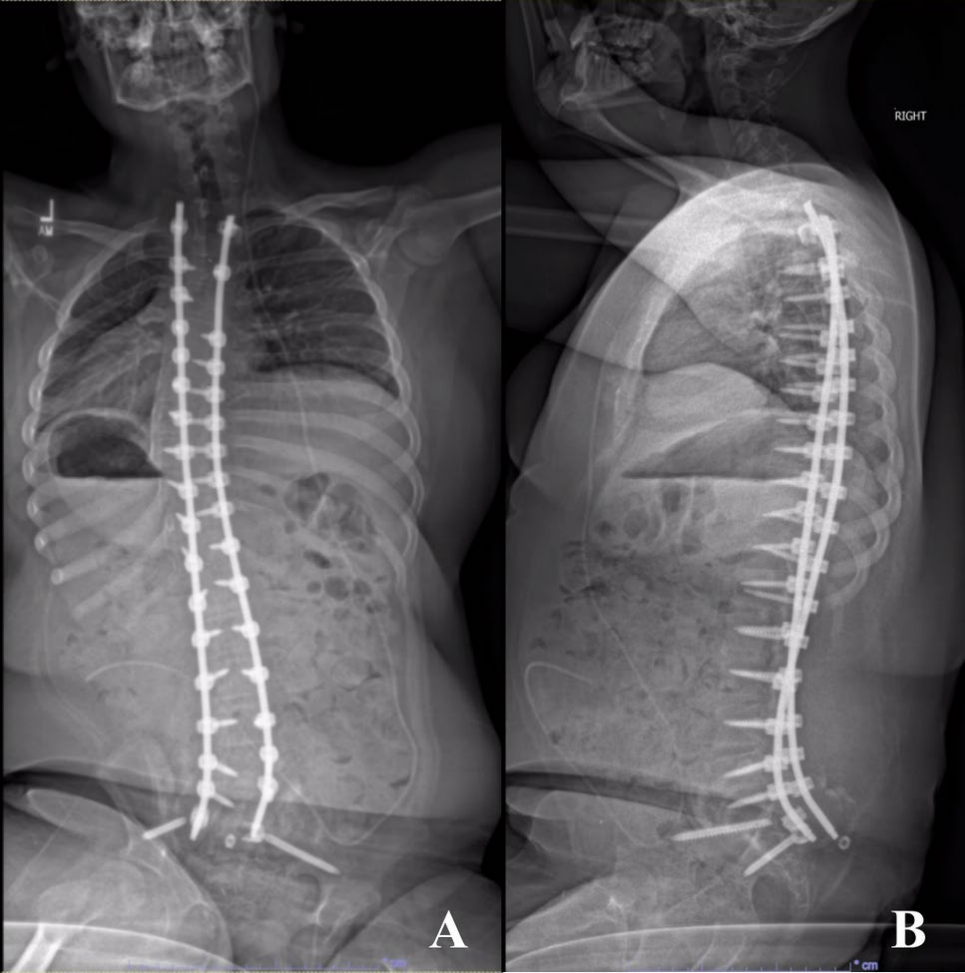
Posteroanterior (A) and lateral (B) sitting spine radiographs of a 15-year-old male with lumbar myelomeningocele treated with T2-Pelvis posterior spinal fusion. Follow-up radiographs at 1 year postoperatively demonstrated set screw dislodgement of the right SAI screw and fracture of the left SAI screw at the head-shaft junction. Both SAI screws were 7.5mm in diameter
